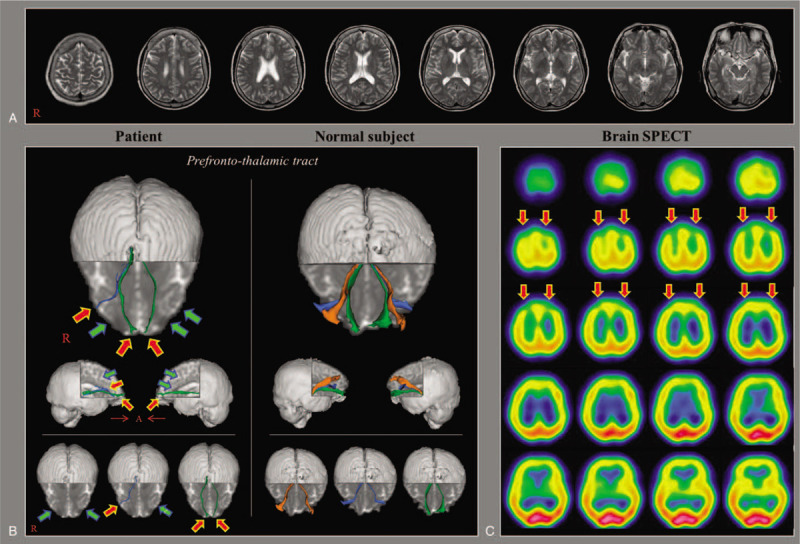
(A) T2-weighted brain magnetic resonance images at 2 months after injury onset show no abnormal lesion. (B) Imaging results for the prefronto-thalamic tracts (PTTs) on diffusion tensor tractography at 2 months after onset compared to that for a normal subject (60-year-old male). Narrowings (red arrows) are observed in the right ventrolateral and both orbitofrontal PTTs, and both the dorsolateral PTTs and the left ventrolateral PTT were not reconstructed (green arows). (C) Brain SPECT (Tc-99m ECD) images show reduction of perfusion in both the frontal lobes, especially in both prefrontal lobes (red arrows).
